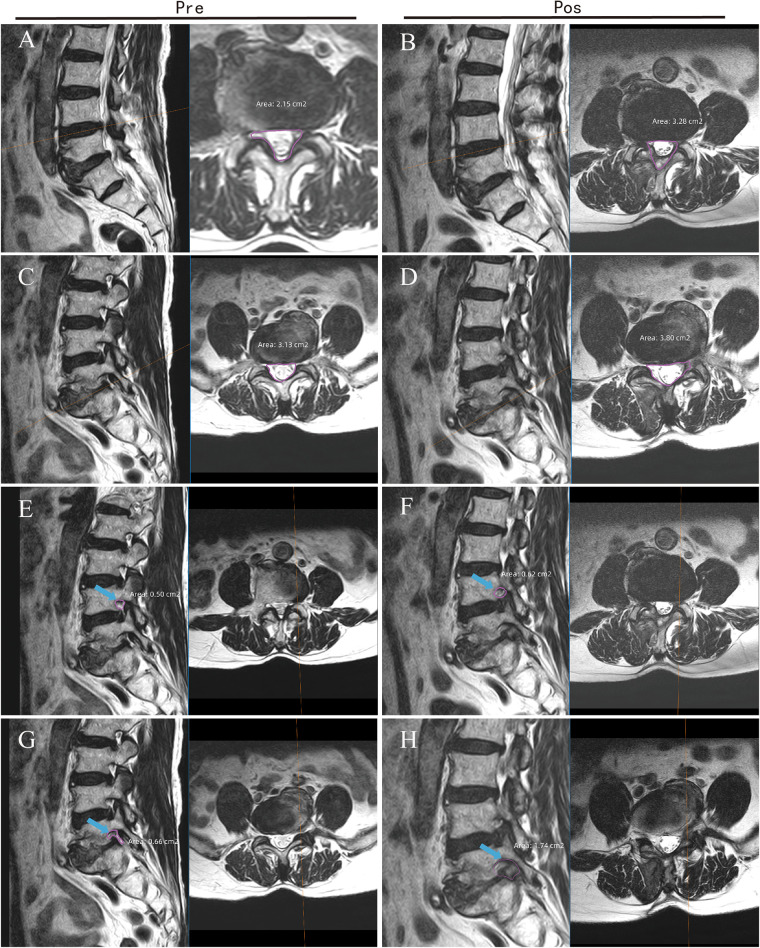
Preoperative vs. postoperative MRI. (A,B) L3-4 sagittal and axial canal areas: preop (A) and postop (B). (C,D) L4-5 sagittal and axial canal areas: preop (C) and postop (D). (E,F) L3-4 sagittal foraminal areas and axial views: preop (E) and postop (F). (G,H) L4-5 sagittal foraminal areas and axial views: preop (G) and postop (H).
